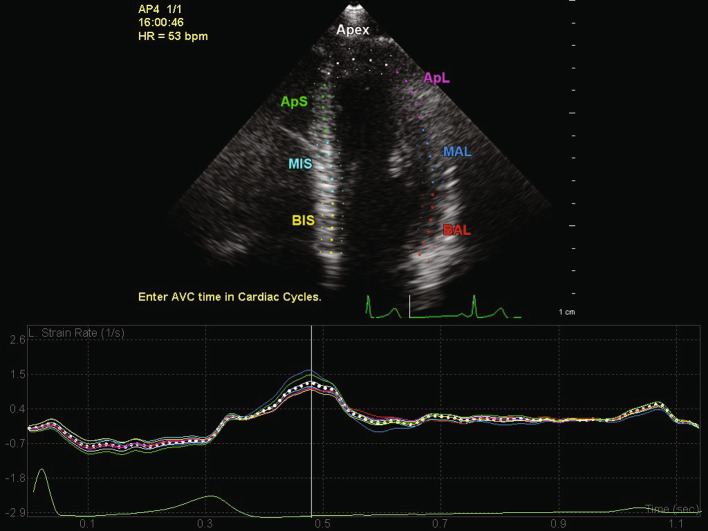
Example of left ventricular (LV) strain measurement in the apical four-chamber view. The colored lines in the graph show LV strain rate measurements for each corresponding segment, the dotted line represents the average strain rate for the all the segments combined
